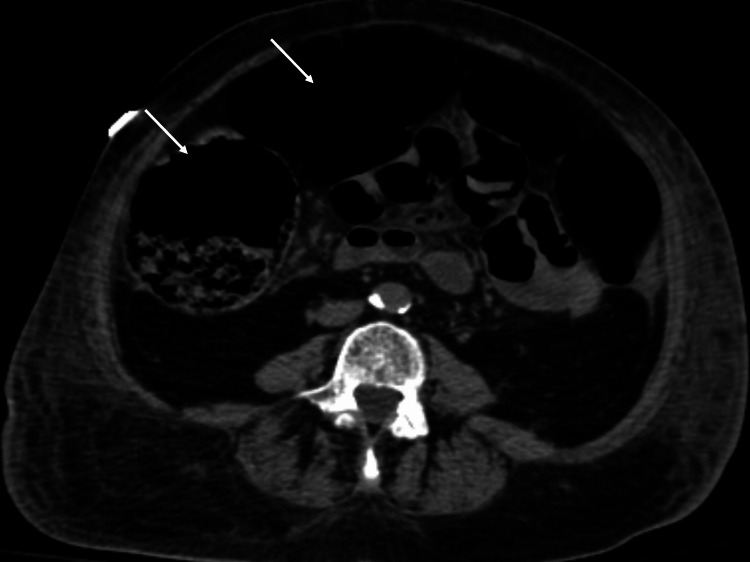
Initial CT scan of the abdomen pelvis without contrast showed non-specific mild distention of the colon (arrows). No finding of small bowel ischemia such as pneumatosis intestinalis, dilated small bowel loops, or portal venous air was noted
